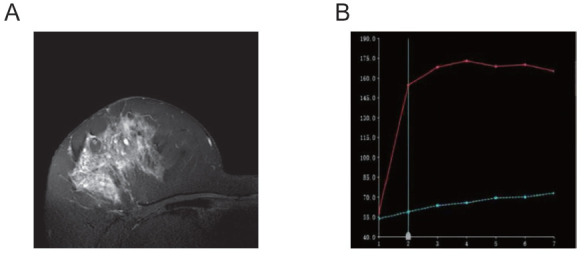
Right breast ductal carcinoma *in situ* (HER2-enriched subtype). The lesion is of the non-mass enhancement (NME) type, with heterogeneous high signal intensity on T2-weighted imaging (A). The TIC shows rapid early enhancement (B).
